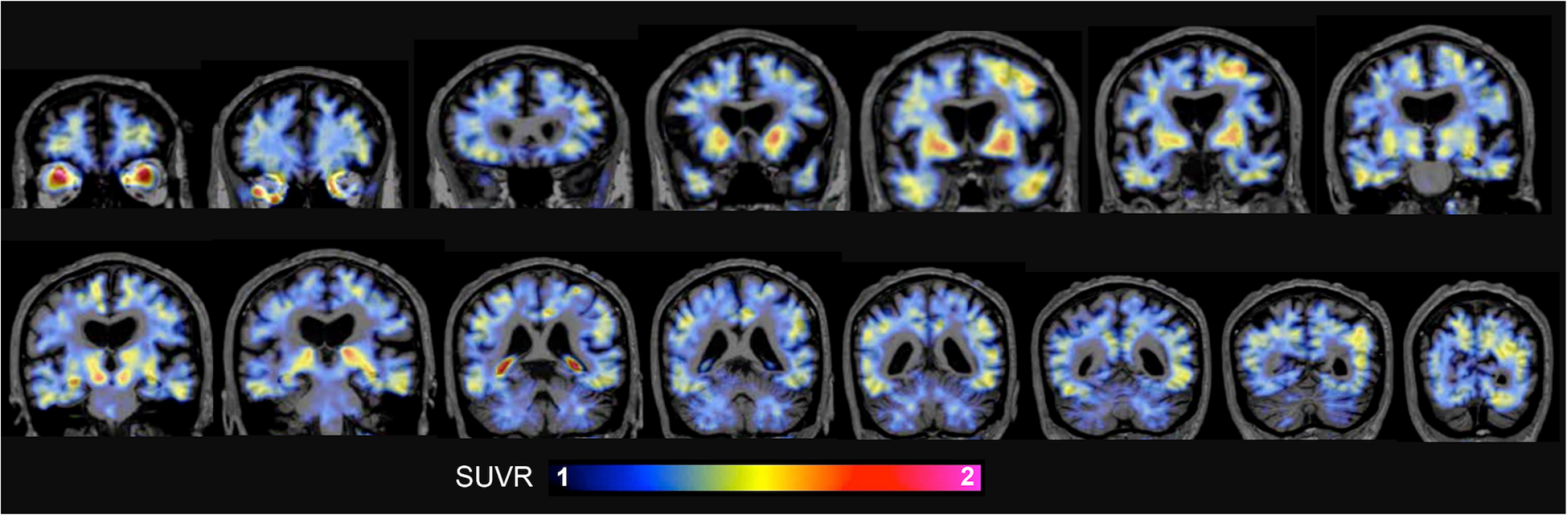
Coronal in vivo [F-18]-AV-1451 PET images of the PD subject. The color scale indicates SUVR range from 1 to 2. The subject exhibited increased tracer retention in basal ganglia, midbrain, choroid plexus and eyeballs, and milder retention in inferior temporal (bilaterally), left middle frontal and left occipital cortices. Abbreviations: PD = Parkinson’s disease; PET = positron emission tomography; SUVR = standardized uptake value ratio
